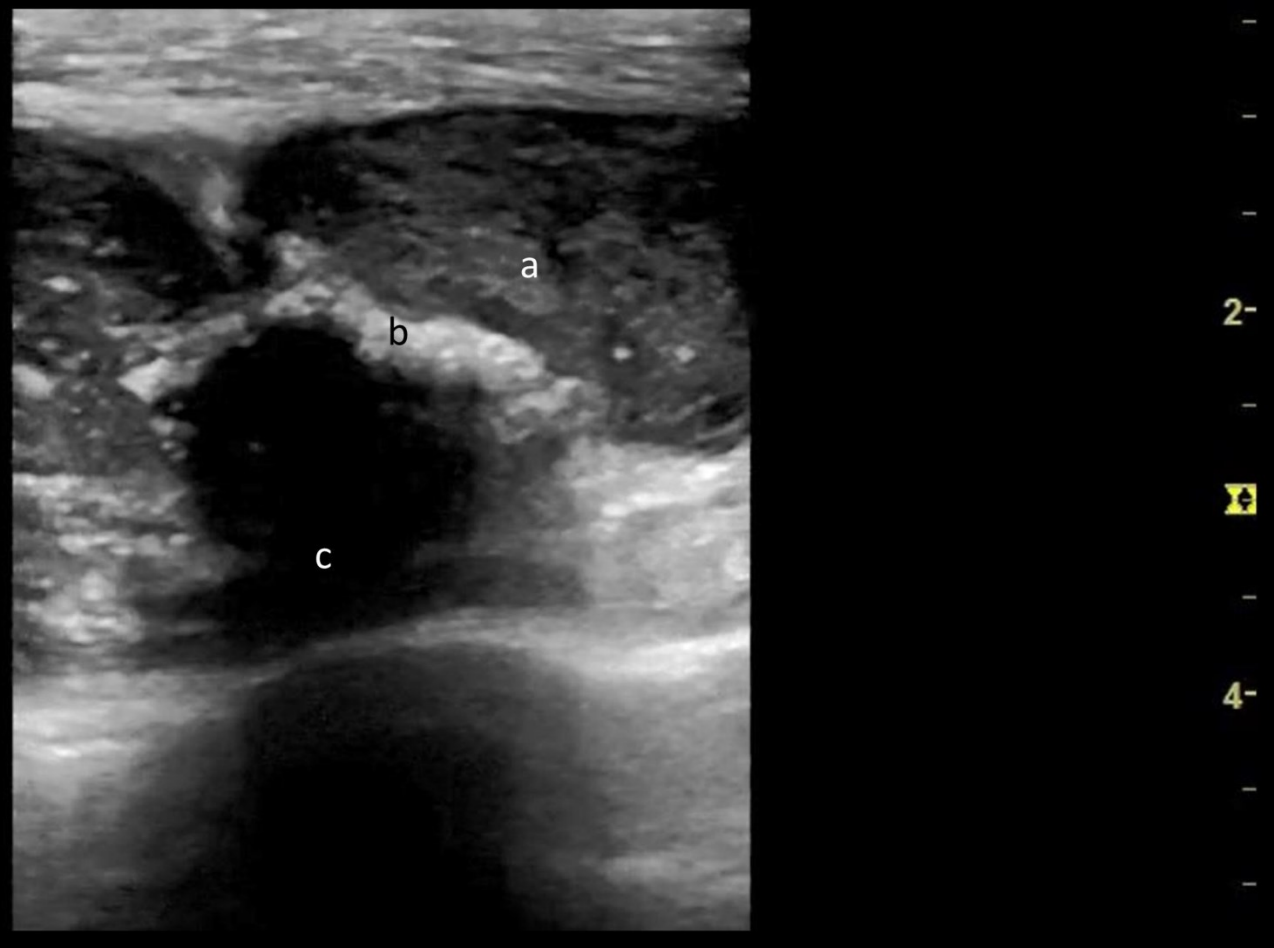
A sonographic image of the cold abscess using a 12 MHz linear probe showing heterogenous fluid collection (a) in the pectoralis major on the left hemithorax. Thickened periosteum of the first rib (b) with break in the cortex representing osteomyelitis. There is posterior acoustic shadowing below the first rib (c).
